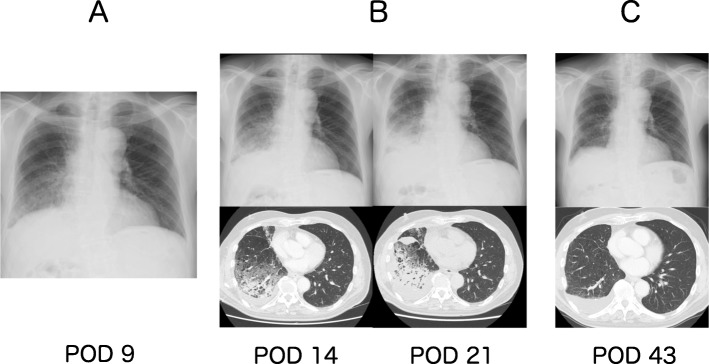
Image findings. a On POD 9, unilateral consolidation appeared on the right lower field. Bacterial pneumonia was thought to have occurred as a common cause of postoperative complication and broad antibiotic therapy using meropenem was started. b After the initiation of meropenem, on POD 14, chest X-ray radiographs and computed tomography scans showed gradually worsening (ground glass, reticular shadows, consolidation, and pleural effusion only on the right operative side). On POD 21, the consolidation on the right side had exacerbated regardless of the administration of meropenem. Thus, we started to question if meropenem itself provoked and worsened the patient’s condition by causing drug-induced AEP. c On POD 43, after cessation of meropenem and use of oral prednisolone, the abnormal shadow in the chest X-ray radiographs and computed tomography scans completely disappeared and the patient responded to treatment
